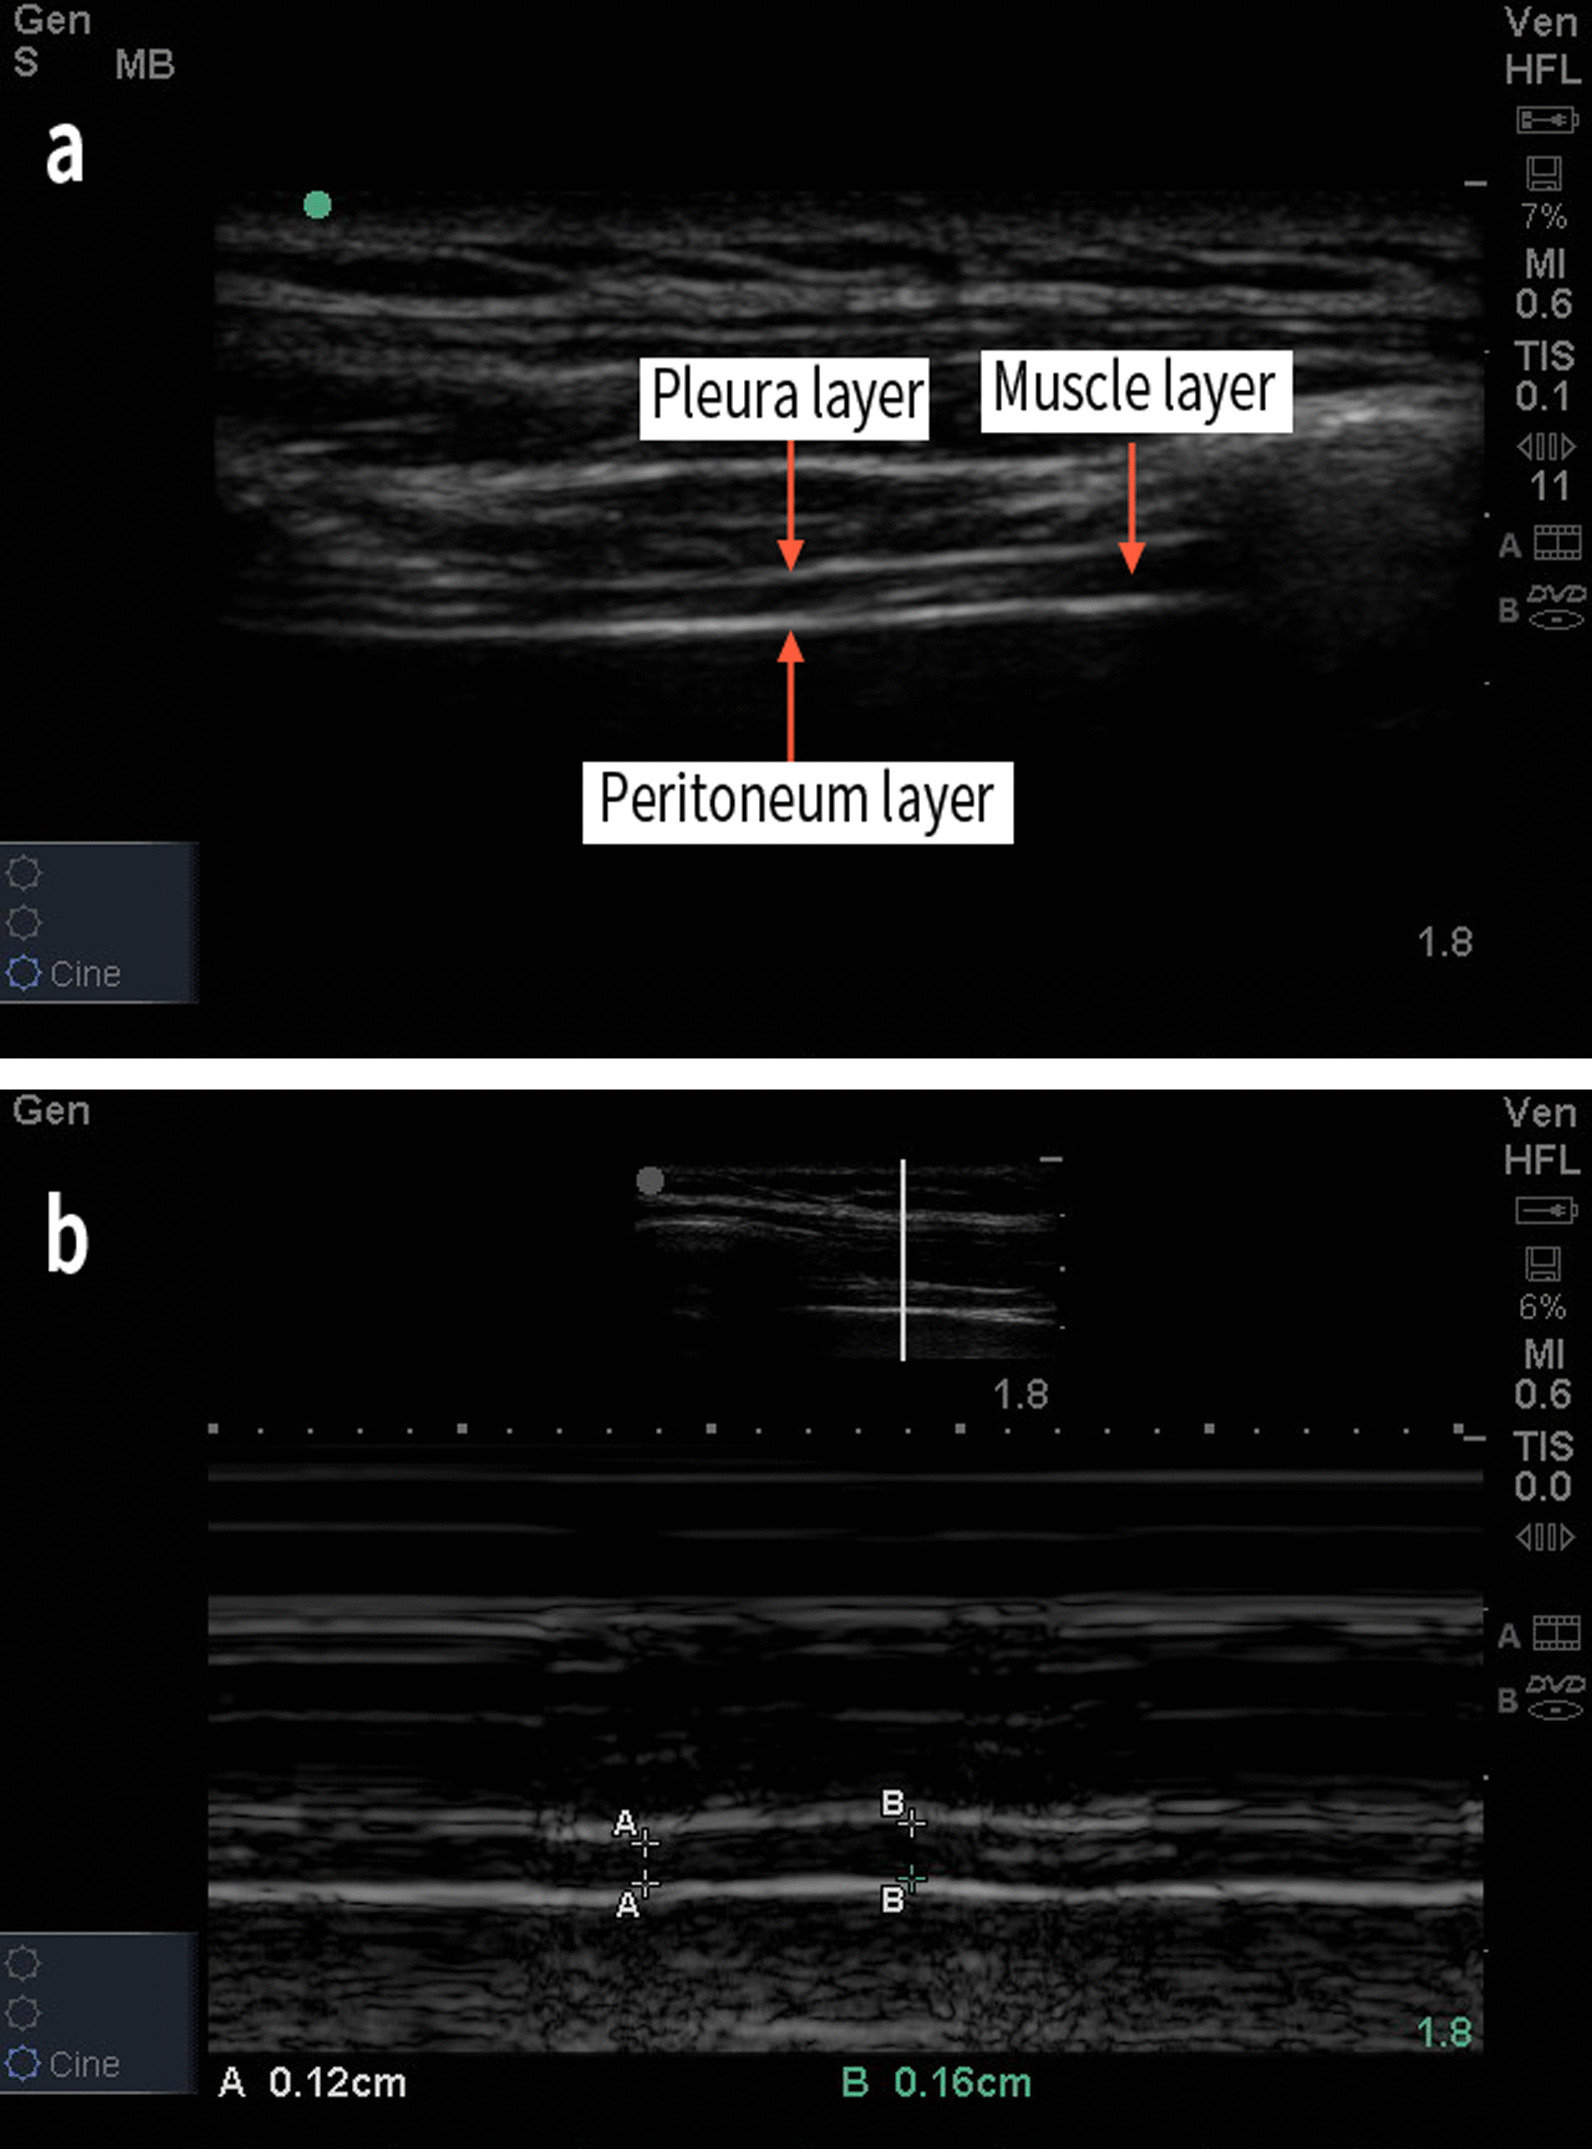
Diaphragm thickness (DT) measurement. a B-mode ultrasonography of the diaphragm in the zone of apposition. DT is a measurement of the muscle layer between the pleural layer and the peritoneal layer. b M-mode ultrasonography of the diaphragm in the zone of apposition. DT is measured at both end-inspiration (B) and end-expiration (A). In this image, diaphragm thickening fraction (DTF) was calculated as: 1.6 − 1.2/1.2 × 100% = 33.3%
